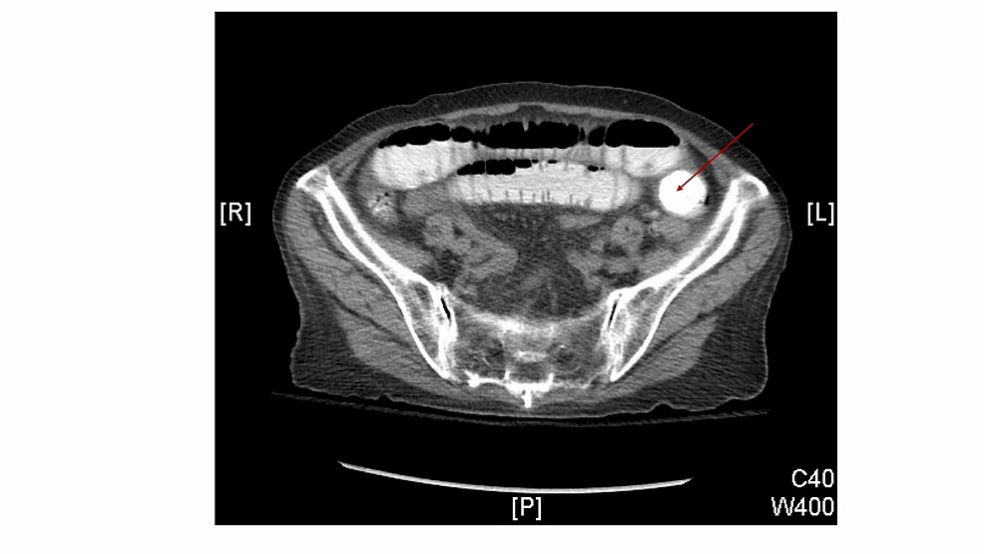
CT scan of the abdomen and pelvis with contrast revealed blockage of the left lower quadrant consistent with gallstone ileus (red arrow) due to a bilioduodenal fistula
